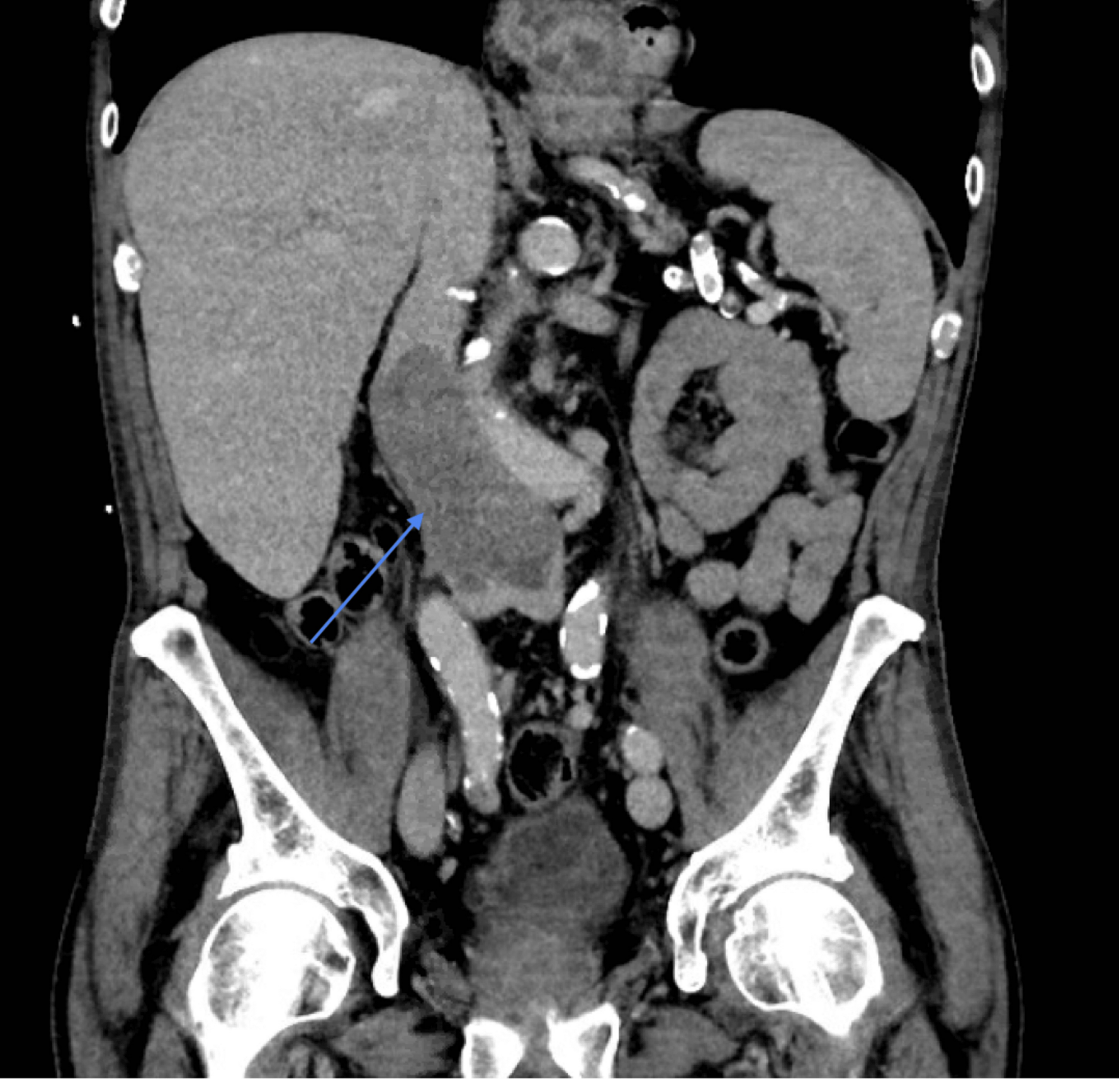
CT abdomen, coronal section. Blue arrow: inferior vena cava (IVC) tumor thrombus.
